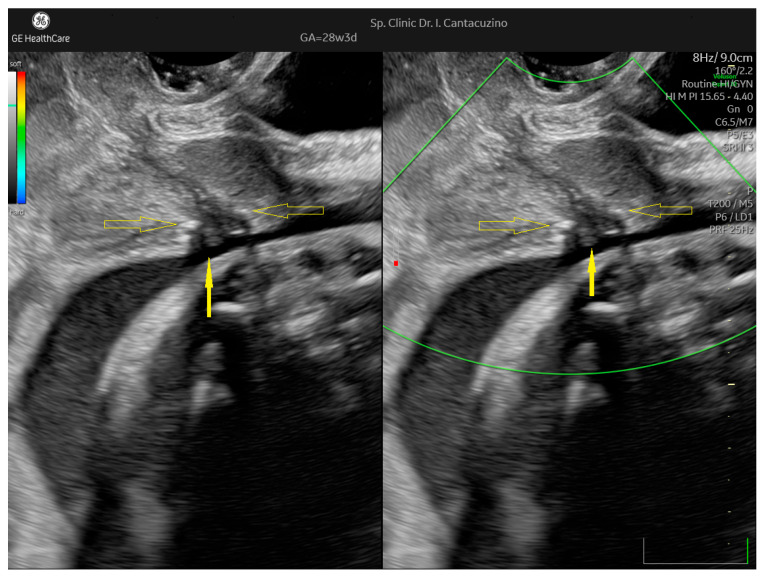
Patient no. 3. Transvaginal ultrasound at 28 W. Sagittal section. Empty arrows point to cerclage thread. Internal distance was 4.1 mm. External distance was 12 mm. Solid arrow points to tendency for funneling below the thread. Dilated vessels were not identified.
